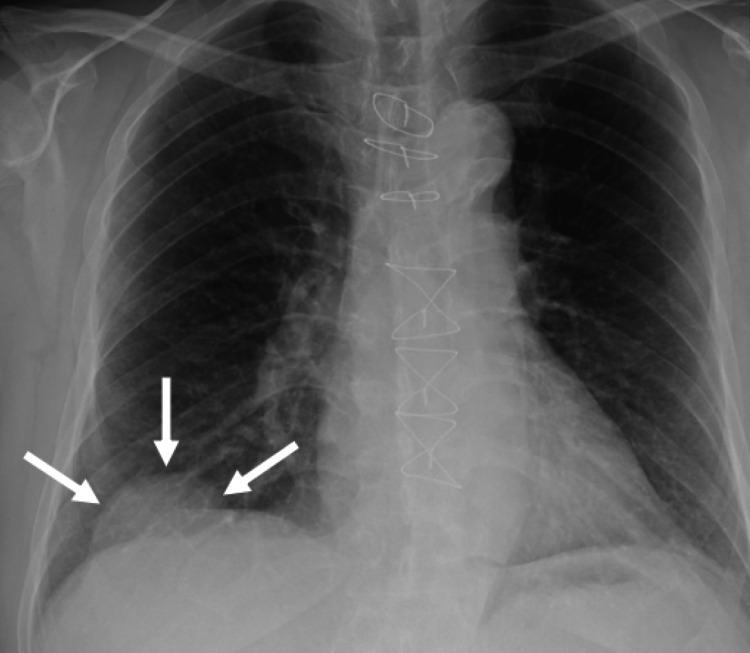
A chest X-ray finding six months after the initial surgery A chest X-ray, six months after initial surgery, showed an abnormal shadow protruding from the hepatic dome (arrows).
